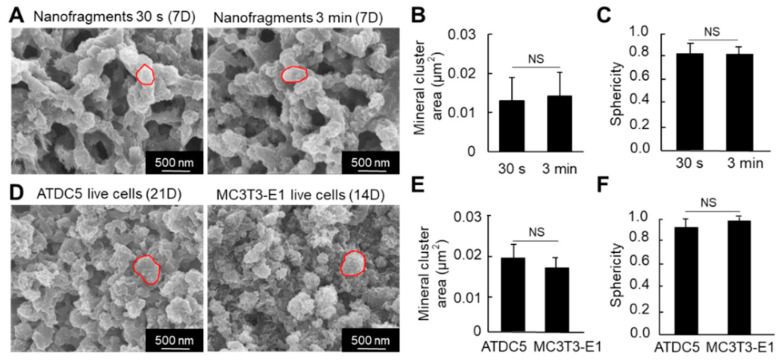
(A) SEM images of the mineral structures formed from cell nanofragment mineralization after treatment with NaClO to remove all organic material. (B,C) Graphs show the mineral cluster area (B) and the sphericity (C) of the minerals formed by the mineralization of cell nanofragments obtained by either 30 s or 3 min of ultrasonication. (D) SEM images of the mineral structures formed from ATDC5 and MC3T3-E1 live cells cultured for 21 and 14 days, respectively, in mineralization-inducing conditions. The minerals were treated with NaClO to remove all organic material. (E,F) Graphs show the mineral cluster area (E) and the sphericity (F) of the minerals formed by the mineralization of ATDC5 and MC3T3-E1 live cells. Note that the size and quasi-spherical shape of the minerals formed from the cell nanofragments were comparable to those formed by ATDC5 and MC3T3-E1 live cells. NS = non-significant, Student’s *t*-test.
